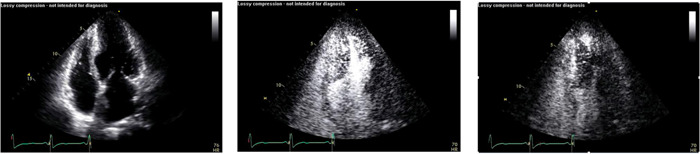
A patient with apical hypertrophic cardiomyopathy which is not well delineated in the unenhanced apical 4‐chamber view (left), well delineated following contrast (center), and demonstrates an apical aneurysm in mid systole (right)
